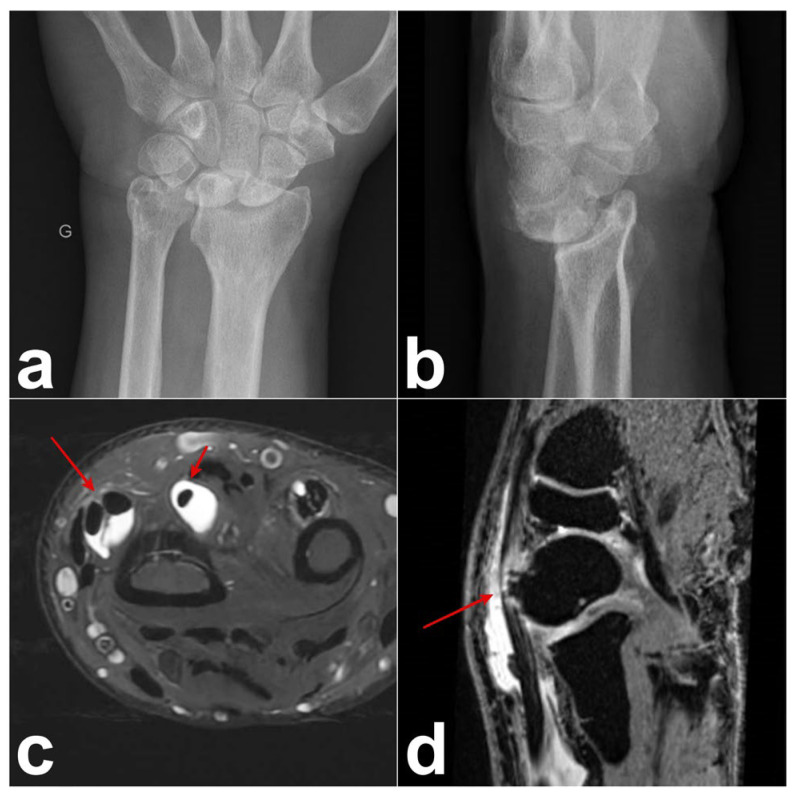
Case 1. (a,b) Preoperative radiograph showing malunion of the distal radius with dorsal subluxation of the wrist as well as radiocarpal and midcarpal arthritis. (c) Wrist MRI with axial T2-weighted fat-saturated gradient-echo sequence. DIT: Tenosynovitis of the EPL, ECRB, and ECRL (red arrows). (d) Wrist MR arthrogram (T1-weighted VIBE (Volumetric Interpolated Breath hold examination [VIBE) sequence with coronal orientation, isotropic resolution, and water excitation): dorsal subluxation of the scaphoid with signs of remodeling and its abrasive action on the ECRB tendon (red arrow).
